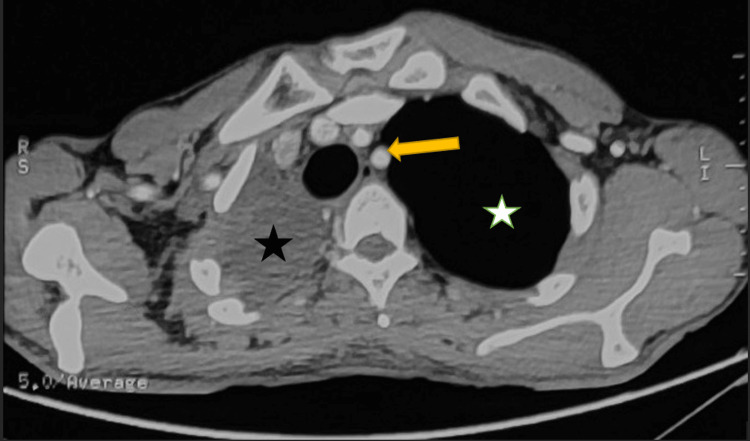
CT chest without contrast (transverse section) at the level of the upper thorax The image shows a moderate right-sided hemothorax (black star) along with a mediastinal and tracheal shift (yellow arrow) to the same side. There is compensatory hyper-expansion of the left lung (white star) CT: computed tomography
